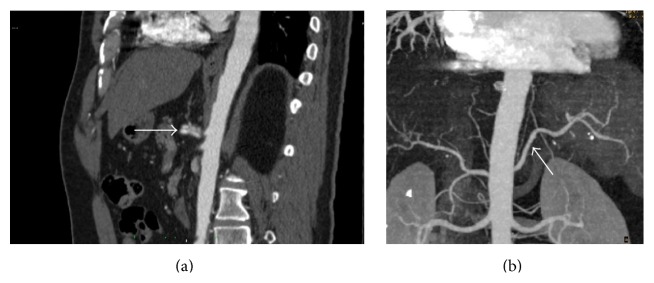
Control CT scan after one month. (a) Sagittal contrast-enhanced CT scan shows ectasia of the celiac artery and stability of intimal dissection compared to the previous scan. (b) 3D reconstruction of the aorta and branch vessels shows patent splenic artery.
